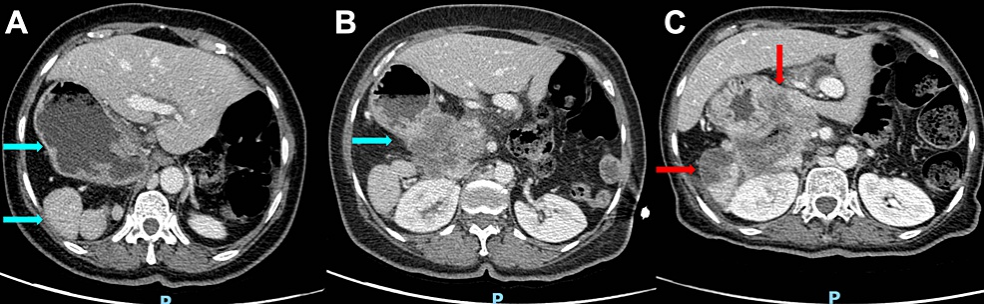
(A) Initial contrast-enhanced CT scan of the abdomen and pelvis (CTAP) revealing the stomach and three splenic tissues located in the right upper quadrant (RUQ). (B) Initial CTAP showing a pancreatic head mass abutting the lesser curvature of the stomach. (C) CTAP four months later showing the previous pancreatic head mass increased in size with new invasion of the spleen in the RUQ.
